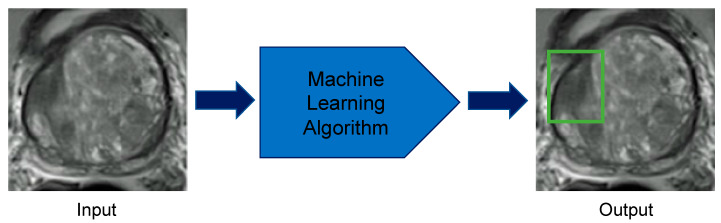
Prostate lesion detection using machine learning methods. The computer takes multiparametric magnetic resonance imaging images of the prostate as inputs and applies the developed machine learning algorithm to correctly localize lesions in the prostate.
